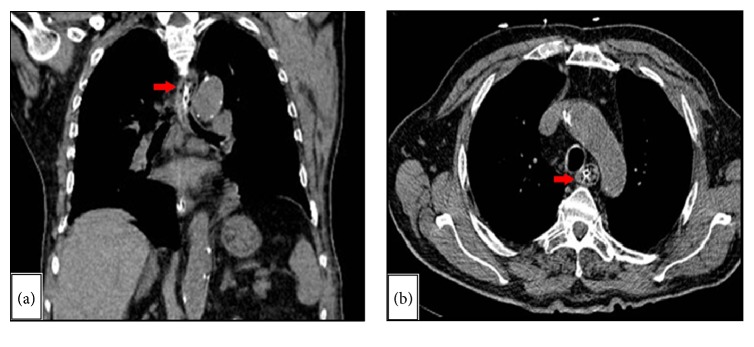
Computed tomography of the chest and upper abdomen ((a) coronal; (b) axial) with minimal administration of contrast through an NG tube, revealing the extent of the bezoar (red arrow) within the upper third of the esophagus.
